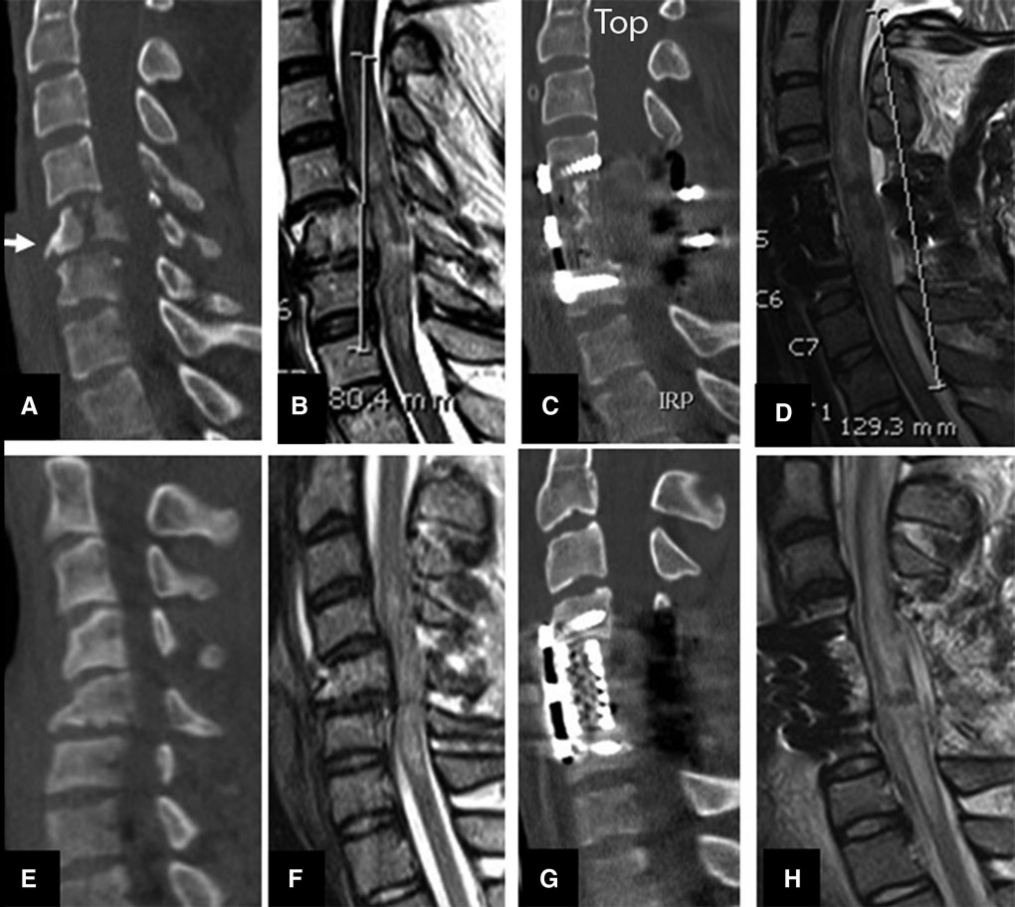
Midsagittal CT and MRI images indicating wo vertical compression and teardrop fractures managed by corpectomy and laminectomy. Upper row plates (A-D) indicate C5 corpectomy and three-level laminectomies and posterior spinal fusion (PSF) with inadequate decompression at C3 and C7. Lower row (E-H) plates showing C5 corpectomy and three-level laminectomy and PSF with full decompression of the spinal cord.
